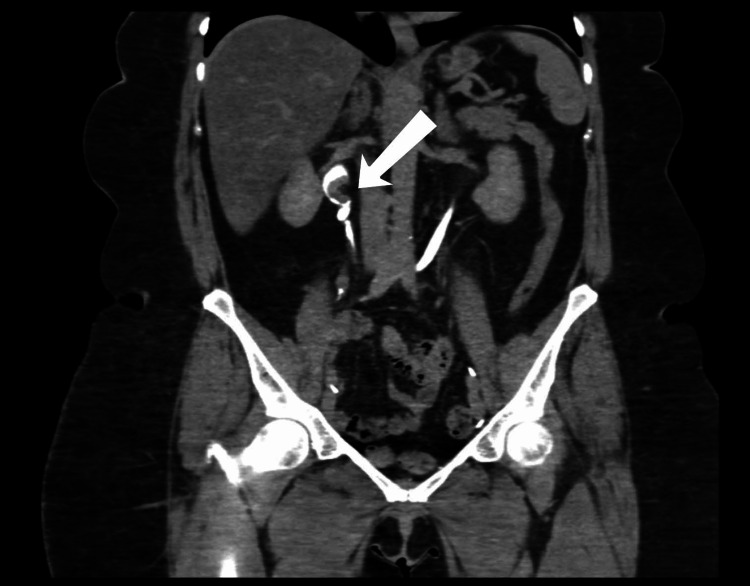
Repeated pelvis and abdomen CT with IV contrast showed a significant mass measured 2 x 1.5 cm. CT: computed tomography; IV: intravenous
